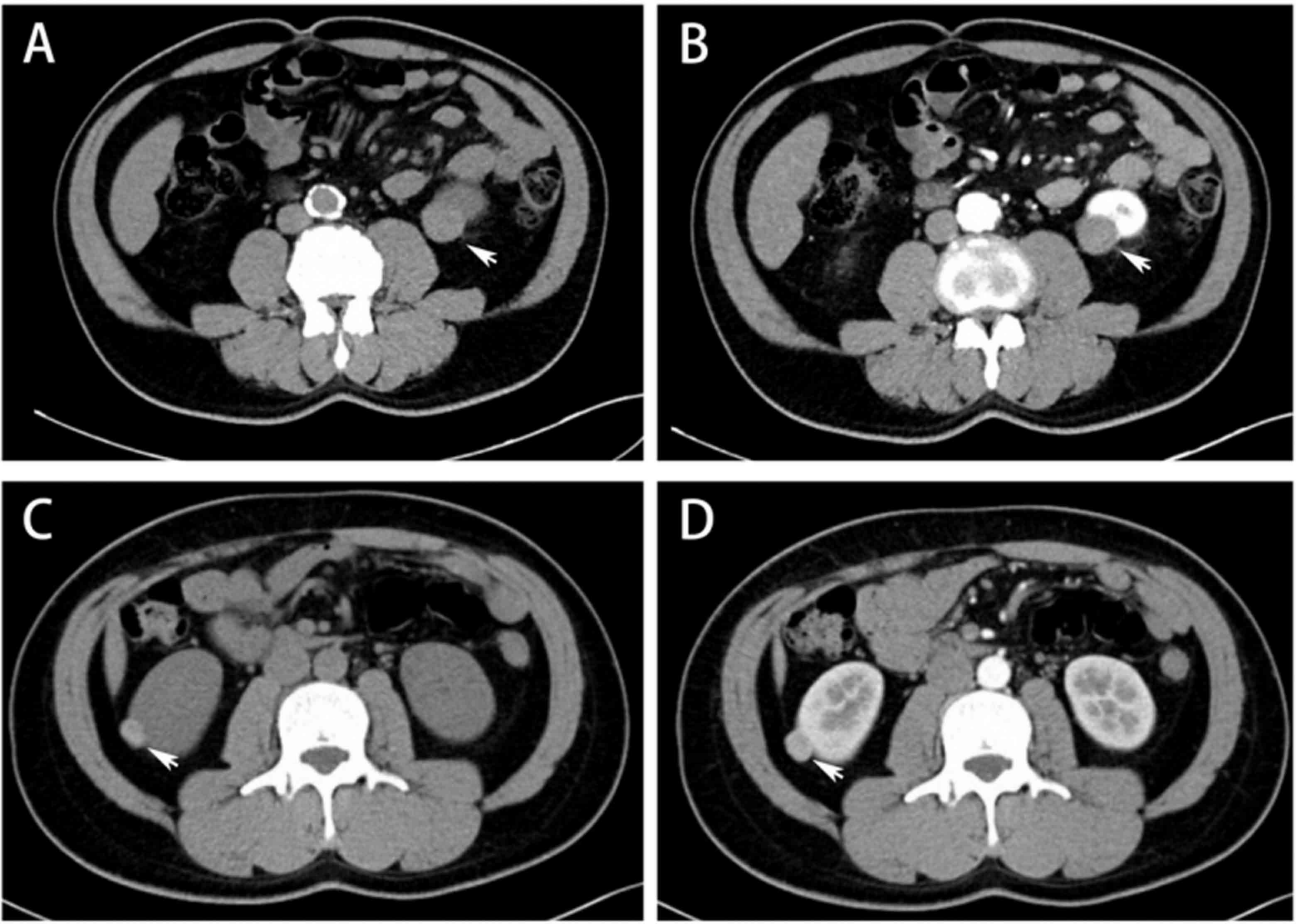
(A, B) Computed tomography revealed a slightly high-density nodule at the lower pole of the left kidney for case one, with a diameter of about 2.5 cm and a CT value of 57 HU on plain scan with no obvious strengthening. (C, D) CT demonstrated a 1.6×1.3 cm round high-density nodule in the right kidney of case 2 with a CT value of about 80HU on plain scan. No enhancement after intravenous administration of contrast was noted.
